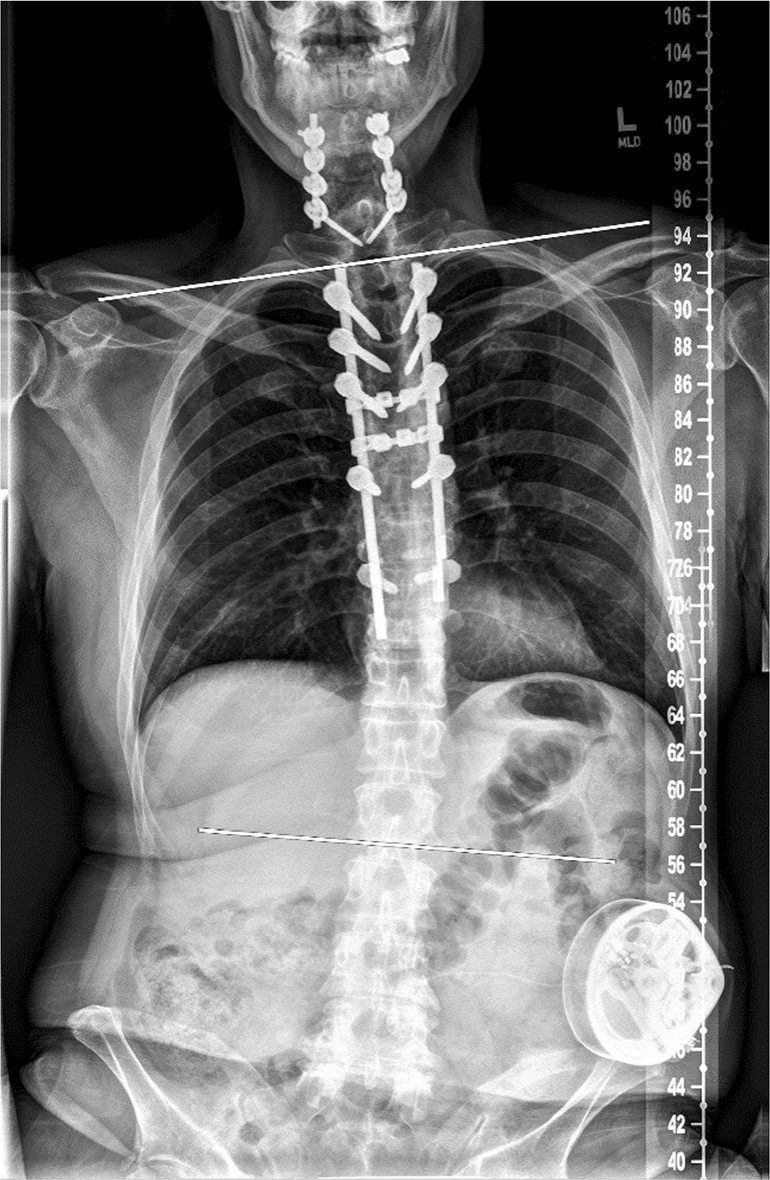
Neuromuscular scoliosis: upright scoliosis radiograph of a 51-year-old male (T6 SCI from a snowboarding injury 18 months earlier) shows neuromuscular dextroscoliosis. The scoliosis angle is 18° measured between the endplates of T2 and L2 (*white lines*). Anteroposterior alignment was normal (not shown)
